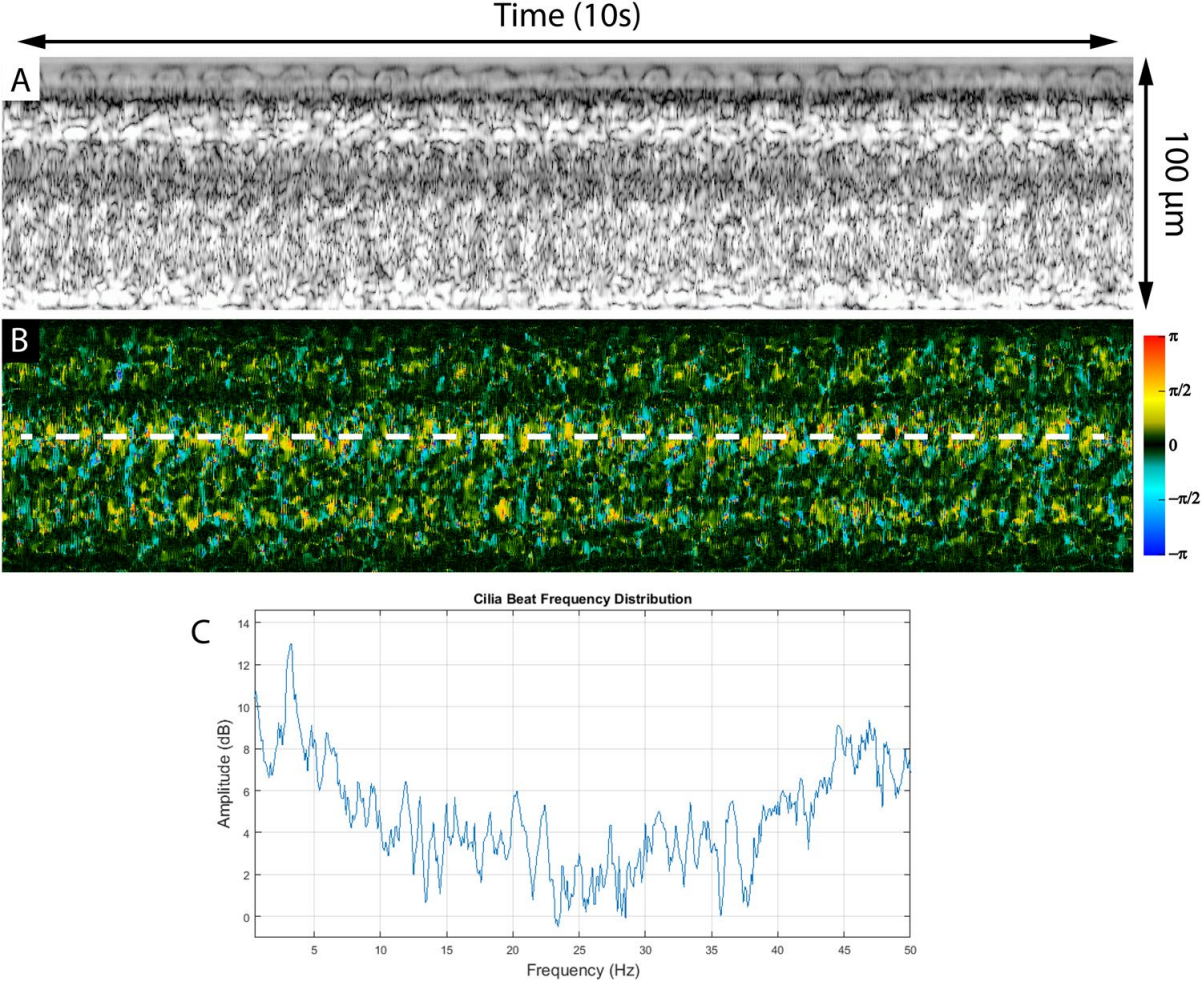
M-mode intensity image at the cilia layer scanned over time (A). Doppler M-mode image over time showing ciliary induced phase shift (B). Fourier transform of phase shift in (B) showing a frequency of 3.8 Hz (C).
